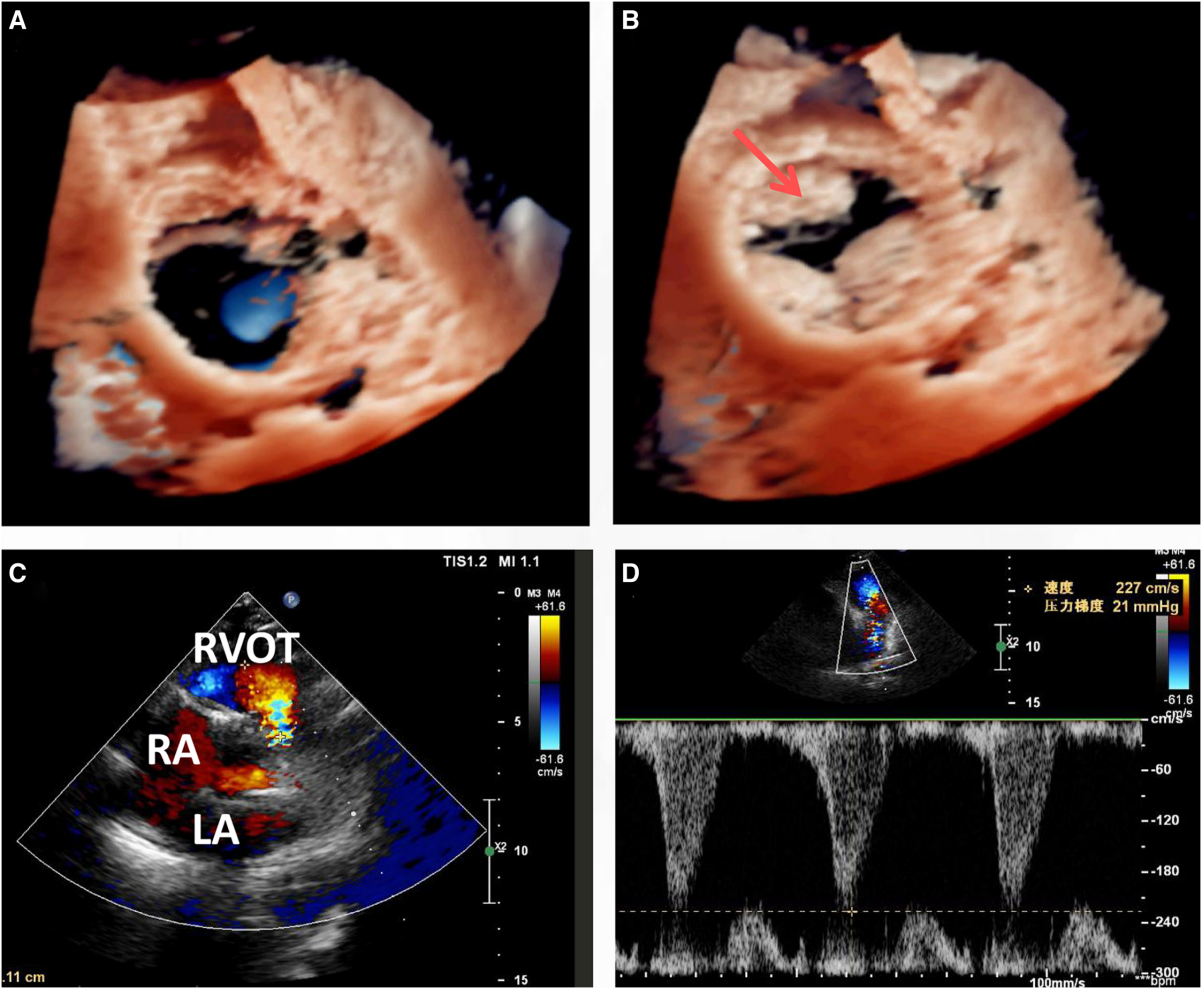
Transthoracic echocardiogram (TTE) at discharge (8 days post-surgery) indicated that the leaflets functioned well in both the cardiac phase of end systolic (A) and diastolic (B), with only mild regurgitation (C,D).
